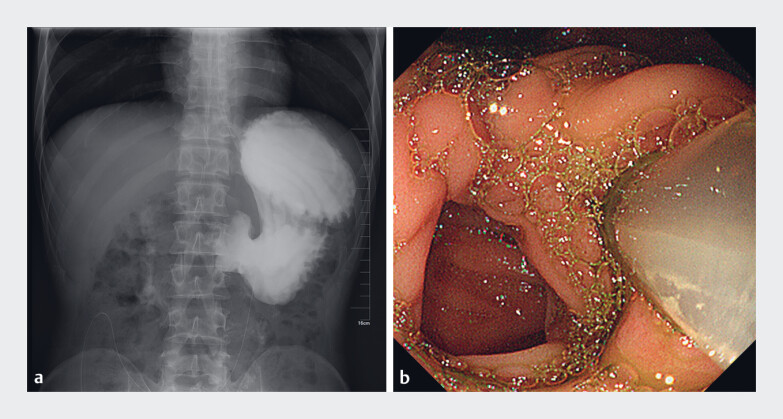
Preoperative iodine-based contrast radiography and gastroscopy.
